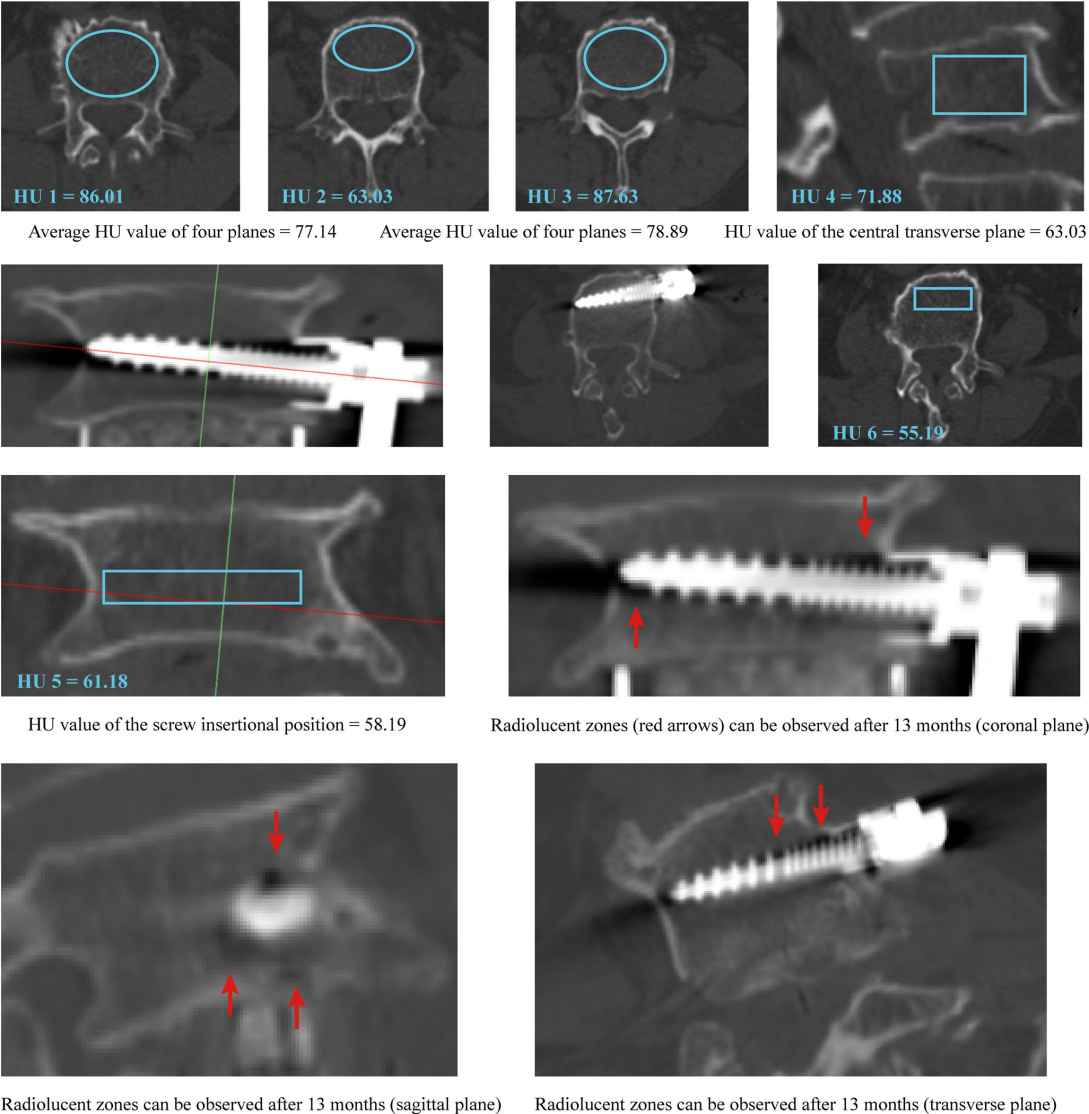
A typical case for the better predictive ability of the screw insertional position’s HU in patients with screw loosening: A 73-year-old female who suffered spinal canal stenosis and was treated by L4-L5 segment OLIF with ALSR fixation. Screw loosening of the cranial vertebral body can be observed in the CT scan at 13 months postoperatively. The HU value measured in the insertional screw position was lower than that measured by the other three methods
